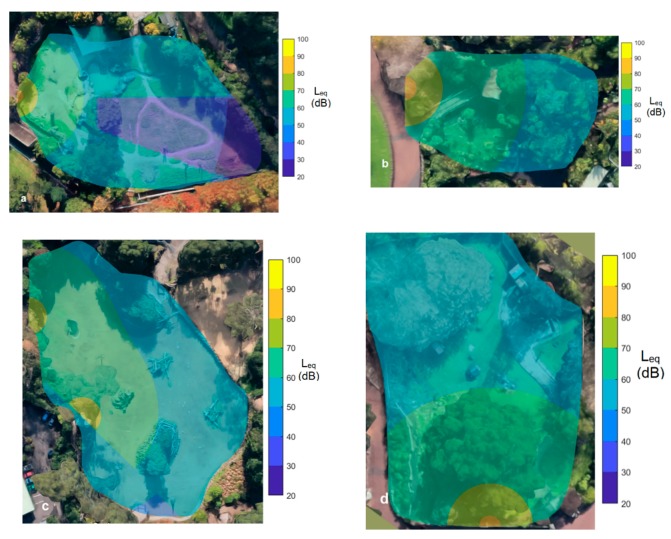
Contours of predicted L_eq_ (dB) at focal species ear heights in the (a) elephant, (b) alligator, (c) giraffe and (d) emu enclosure. (Imagery ©2019 Google, MapData Sciences Pty Ltd., PSMA).
